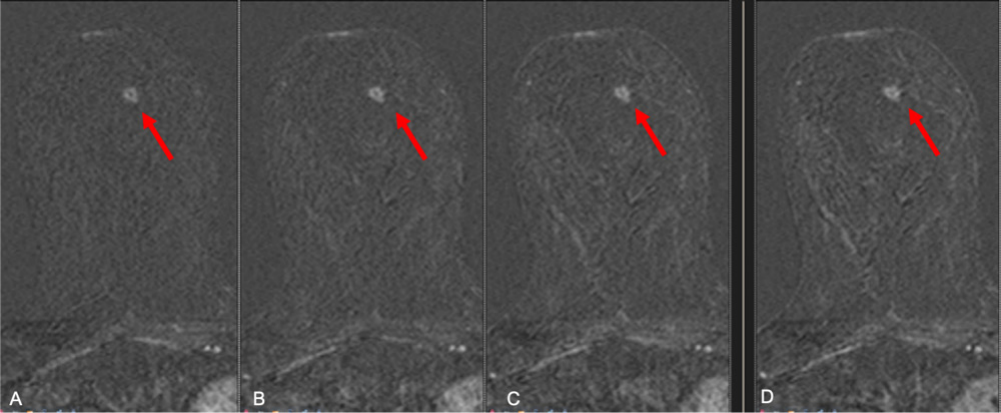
MRI of the right breast. *T*_1_weighted fat sat subtracted at 2 min (A), 4 min (B), 6 min (C) and 8 min (D) after administration of contrast media. There is a 9 mm non-mass enhancing lesion, with in-homogenous uptake of contrast media which is persistent overall the dynamic phase.
